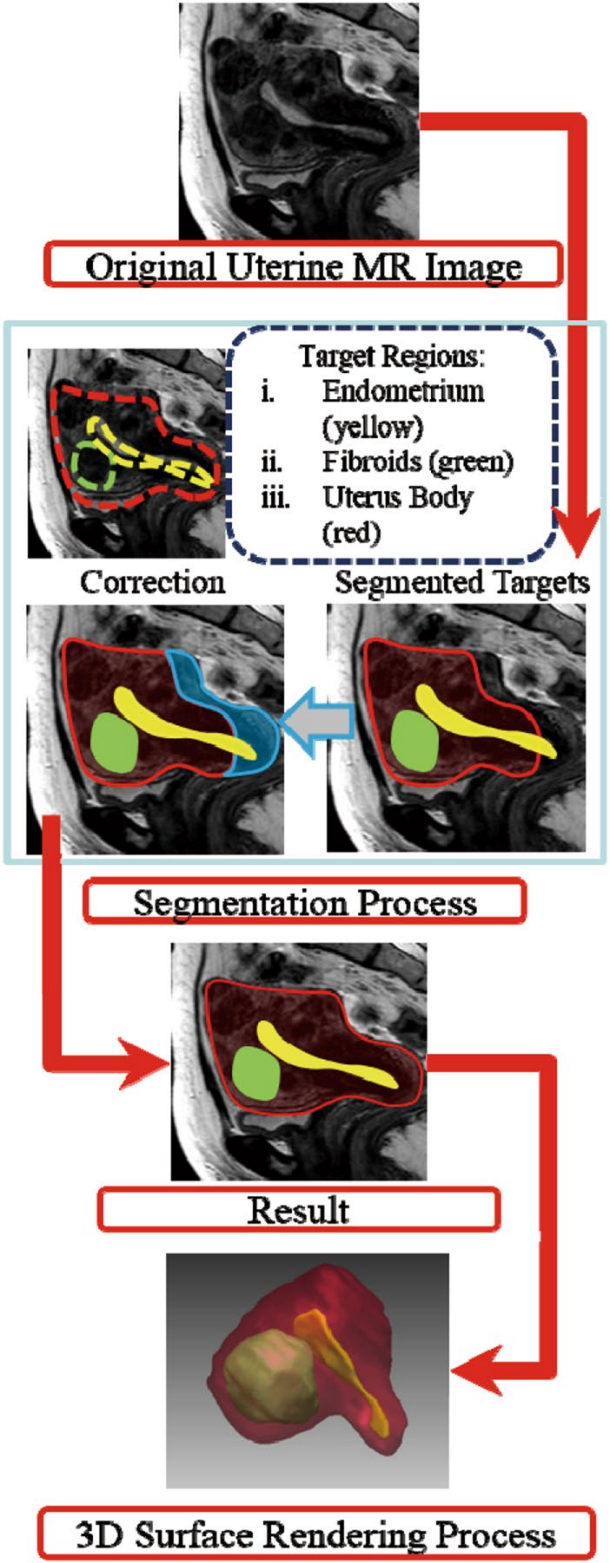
Process flow of the segmentation and 3D rendering. First, the target area (endometrium, fibroids, and uterine body) of the uterine MR images was segmented. Then, a correction process was applied to each slice from different image directions. Finally, a 3D surface rendering visualization was created.
